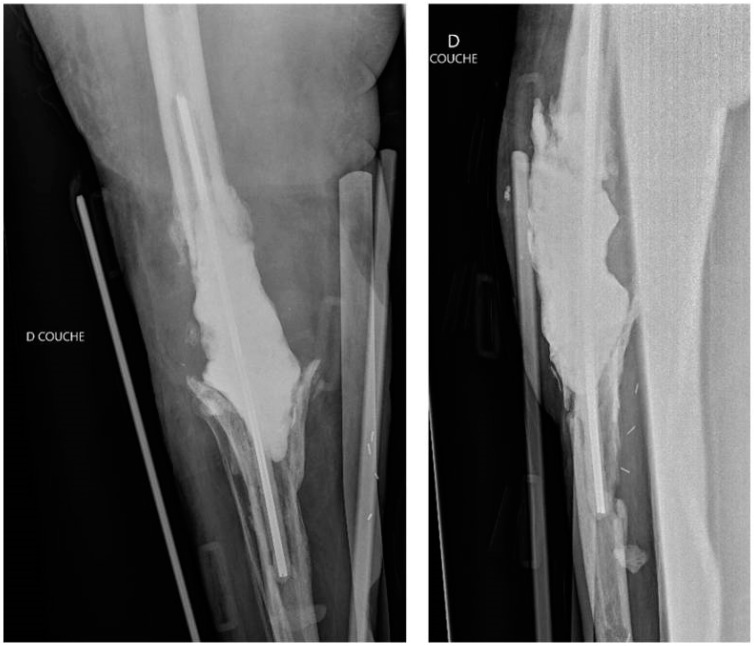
An example of a hand-made static antibiotic-loaded cemented spacer in a case of severe periprosthetic joint infection. Anteroposterior and lateral post-operative radiographic views of the right knee after removal of the infected implants and placement of a cemented spacer loaded with vancomycin and gentamycin, fixed with two metal rods [50]. [Authors’ previously published work, used under the terms and conditions of the Creative Commons Attribution (CC BY) license (https://creativecommons.org/licenses/by/4.0/, accessed on 10 August 2024).].
